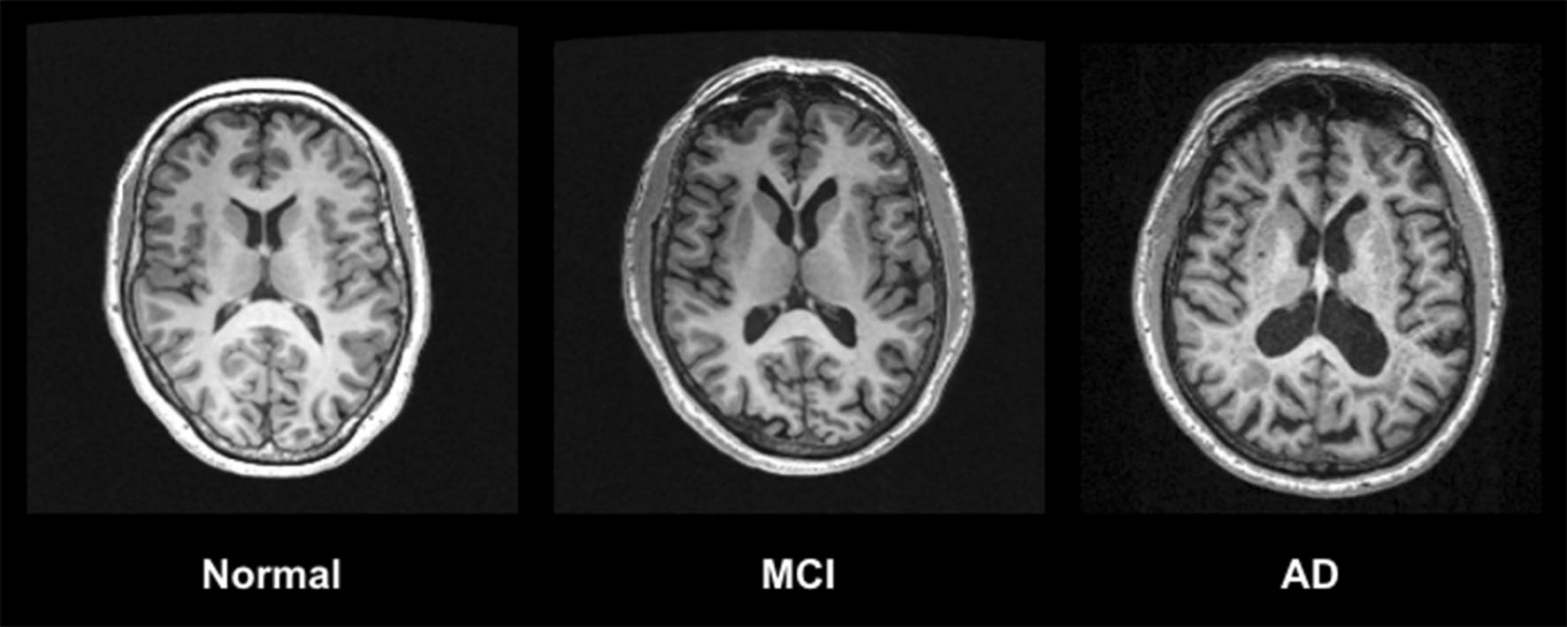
T1-weighted MRI imaging using an MPRAGE (Magnetisation Prepared Rapid Gradient Echo) sequence shows decreased GM volume in an AD patient compared to a healthy control and intermediate GM decline in a patient with MCI
